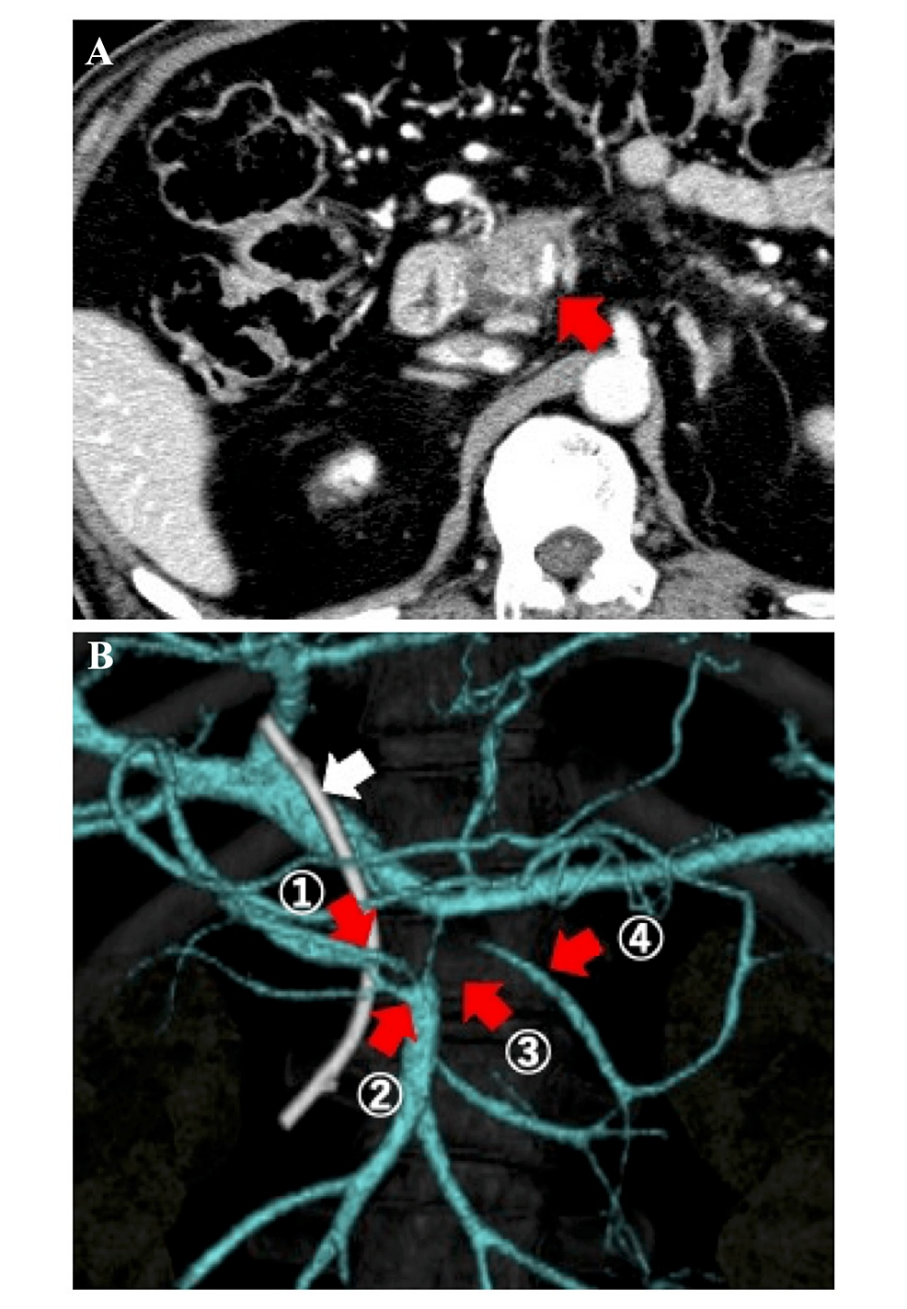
Computed tomography (CT) and three-dimensional CT findings (A) Tumor of 3.0 cm located in the pancreatic head and suspected superior mesenteric vein (SMV) invasion (red arrow). (B) Stenosis by tumor invasion. The red arrow indicates the ① middle colic vein, ② gastrocolic trunk, ③ superior mesenteric vein, and ④ inferior mesenteric vein. The white arrow indicates the endoscopic retrograde biliary drainage tube.
